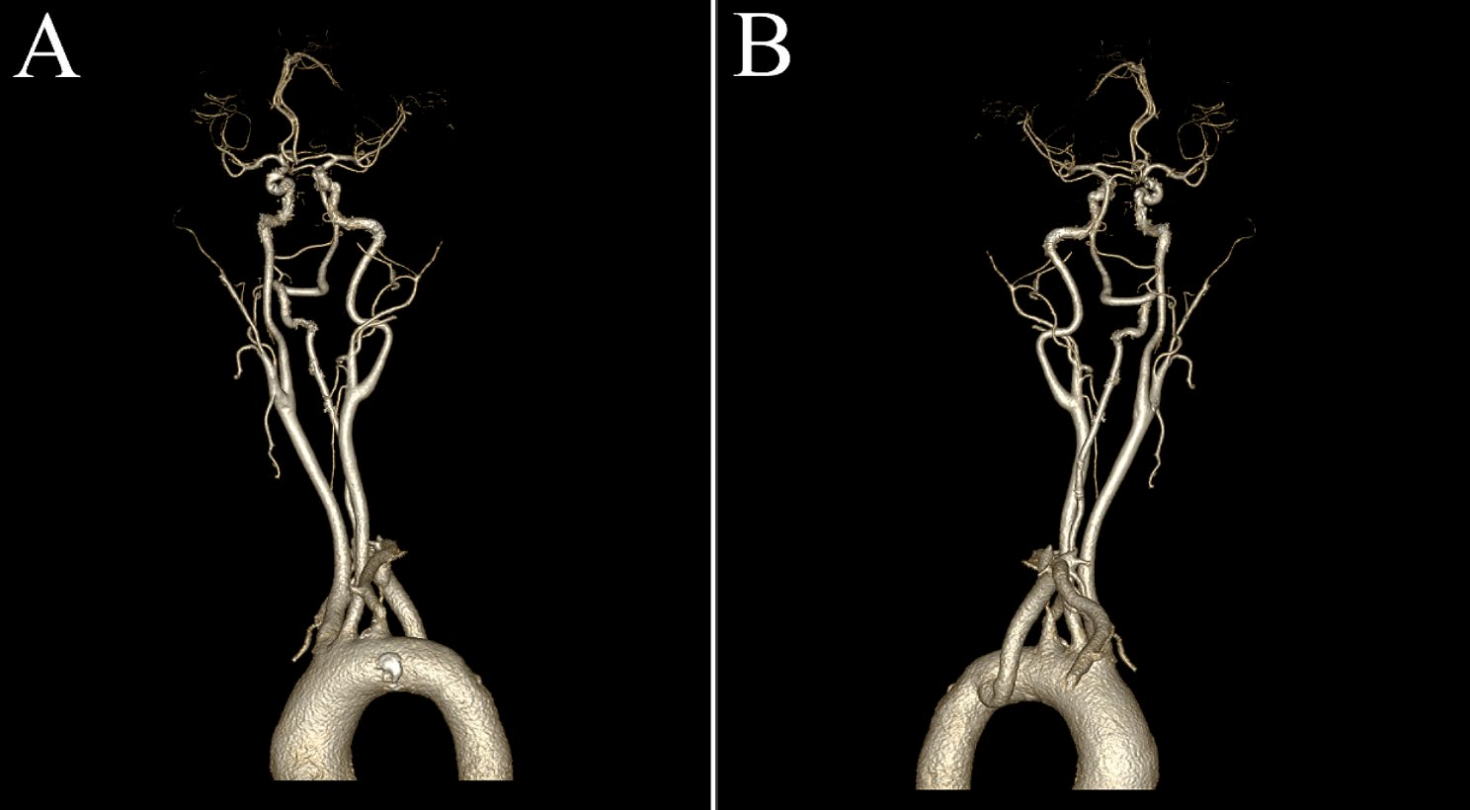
Follow-up computed tomography angiography reveals no stenosis at the compression site of the right vertebral artery (A Anterior view; B Lateral view)
